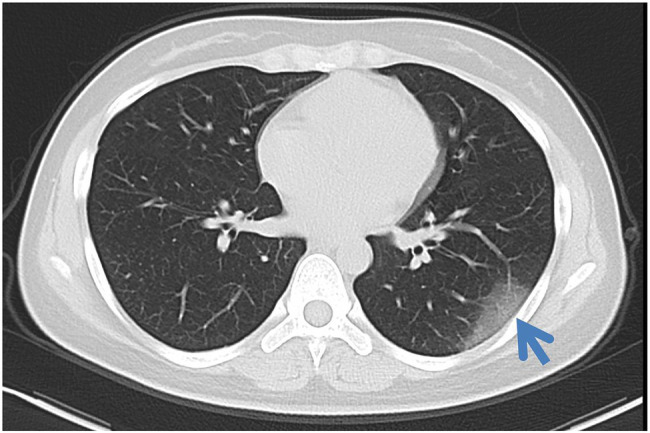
Axial non-contrast-enhanced CT image from a 26-year-old female patient with COVID-19. Pure ground-glass opacities were observed in the peripheral area in the left lower lobe. The maximum diameter of the lesion was 4.5 cm. The left lower lobe score was 1 because the lung parenchyma was less than 25%.
